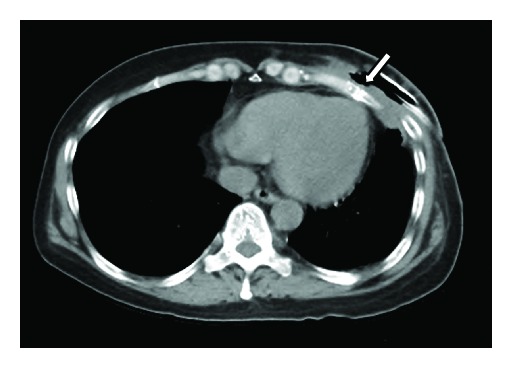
Computed tomography showing that the abscess cavity has shrunk and that the fracture in the fifth rib has healed (arrow).
